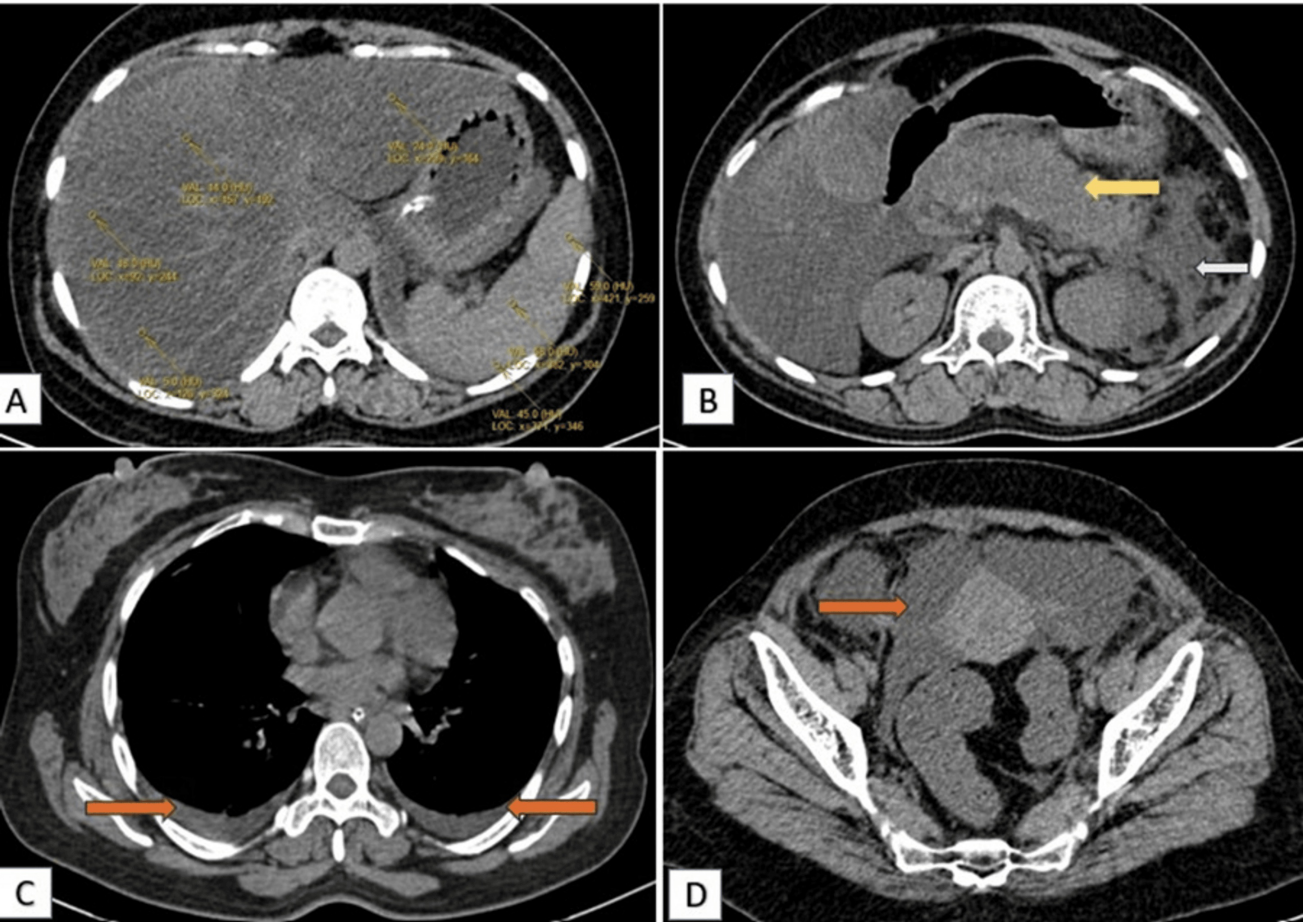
Axial plain CT images depicting changes in fatty liver with acute pancreatitis (A) Axial plain CT images depict relative hypoattenuation of the liver, liver attenuation less than the spleen (changes of fatty liver). (B) The pancreas appears bulky (yellow arrow) with peripancreatic fat stranding and reactive thickening of the left lateral conal fascia (white arrow). (C) Bilateral pleural effusion (orange arrow). (D) Free fluid seen in the pelvis region (orange arrow). CT: computed tomography
